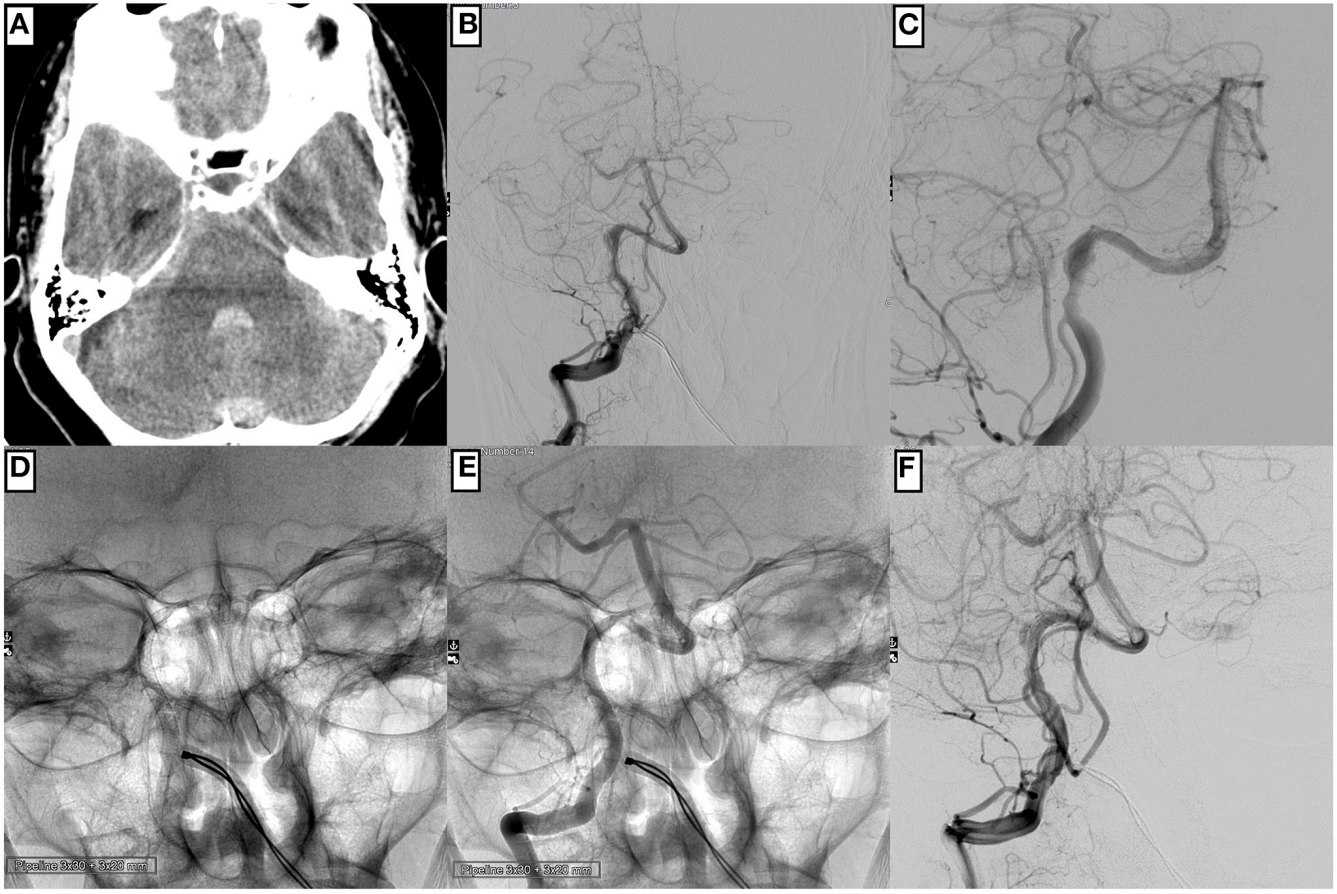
An example of technically unremarkable PED implantation is to reconstruct a right-hand side distal V4 segment affected by an extensive dissecting aneurysm in a 58-year-old male patient. (A) Cranial computed tomography prior intervention displays SAH Fisher grade 3. The injection of the right-hand side vertebral artery in posterior–anterior (B) and working projection (C) demonstrates an extensive, hourglass-shaped dissecting aneurysm directly distal to the PICA orifice. After uneventful catheterization with a Phenom™ 27 microcatheter, two PED flow diverters are implanted in telescoping fashion (D,E). The control injection demonstrates timely opacification of the PICA and the distal vertebrobasilar vessels together with delayed and prolonged opacification of the pseudoaneurysm (F).
